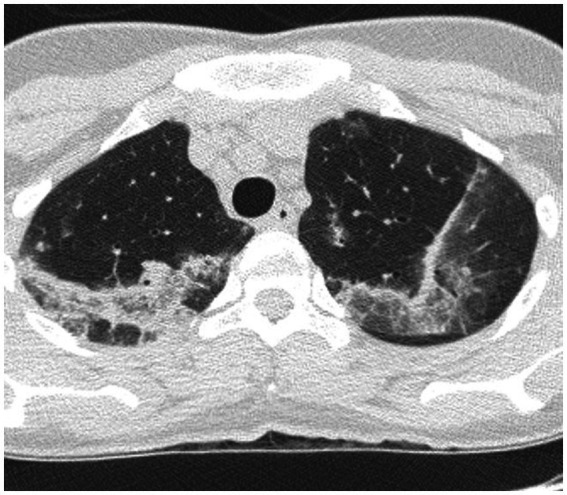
HRCT findings in acute eosinophilic pneumonia. Bilateral patchy areas of ground-glass opacity in both lungs and interlobular septal thickening.
